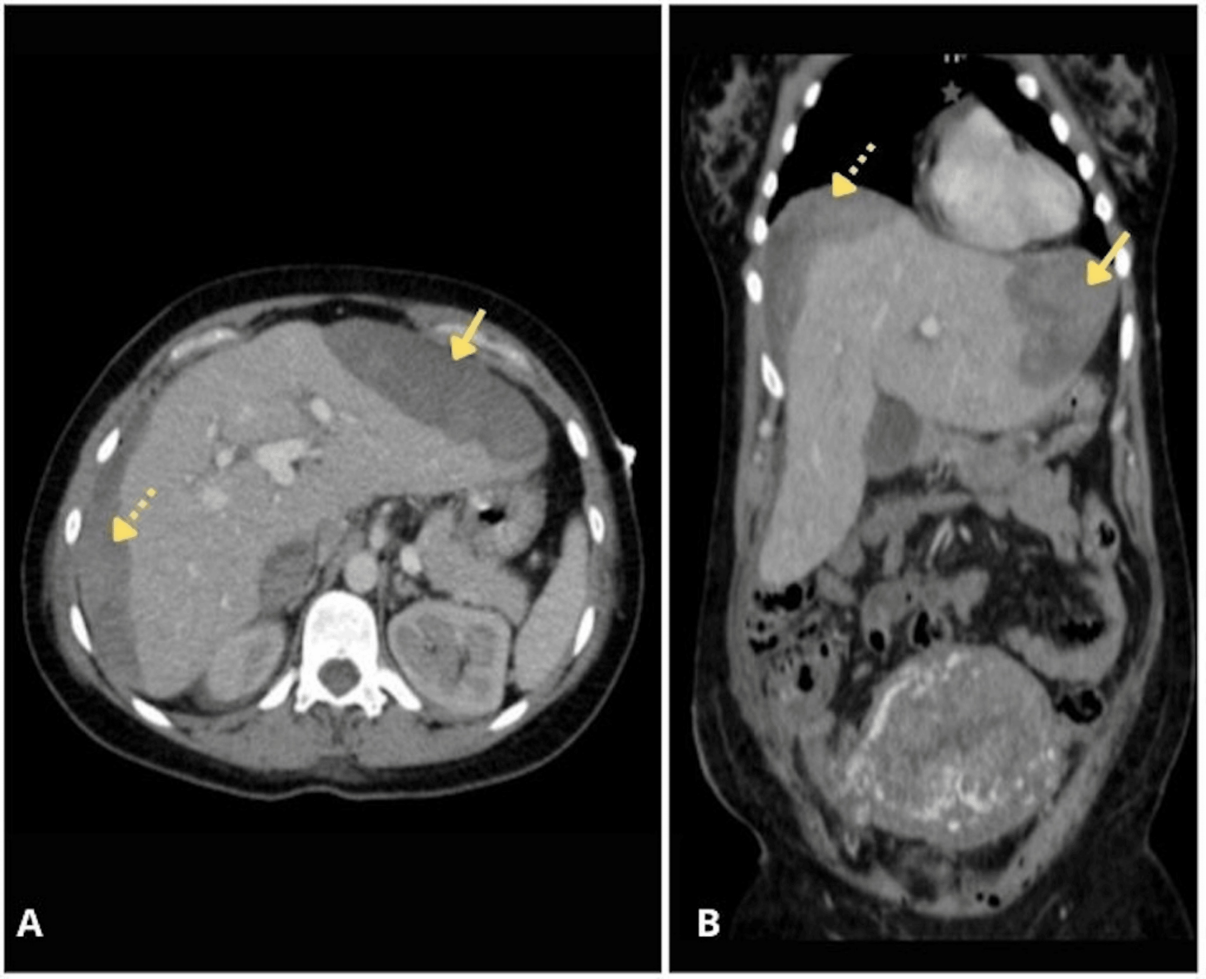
Baseline CT imaging (contrast-enhanced). A: Axial view showing large right-lobe subcapsular hematoma (dotted arrow) and smaller left-lobe lesion (solid arrow). B: Coronal reconstruction demonstrating bilateral involvement, total estimated volume ≈1,790 cc.
